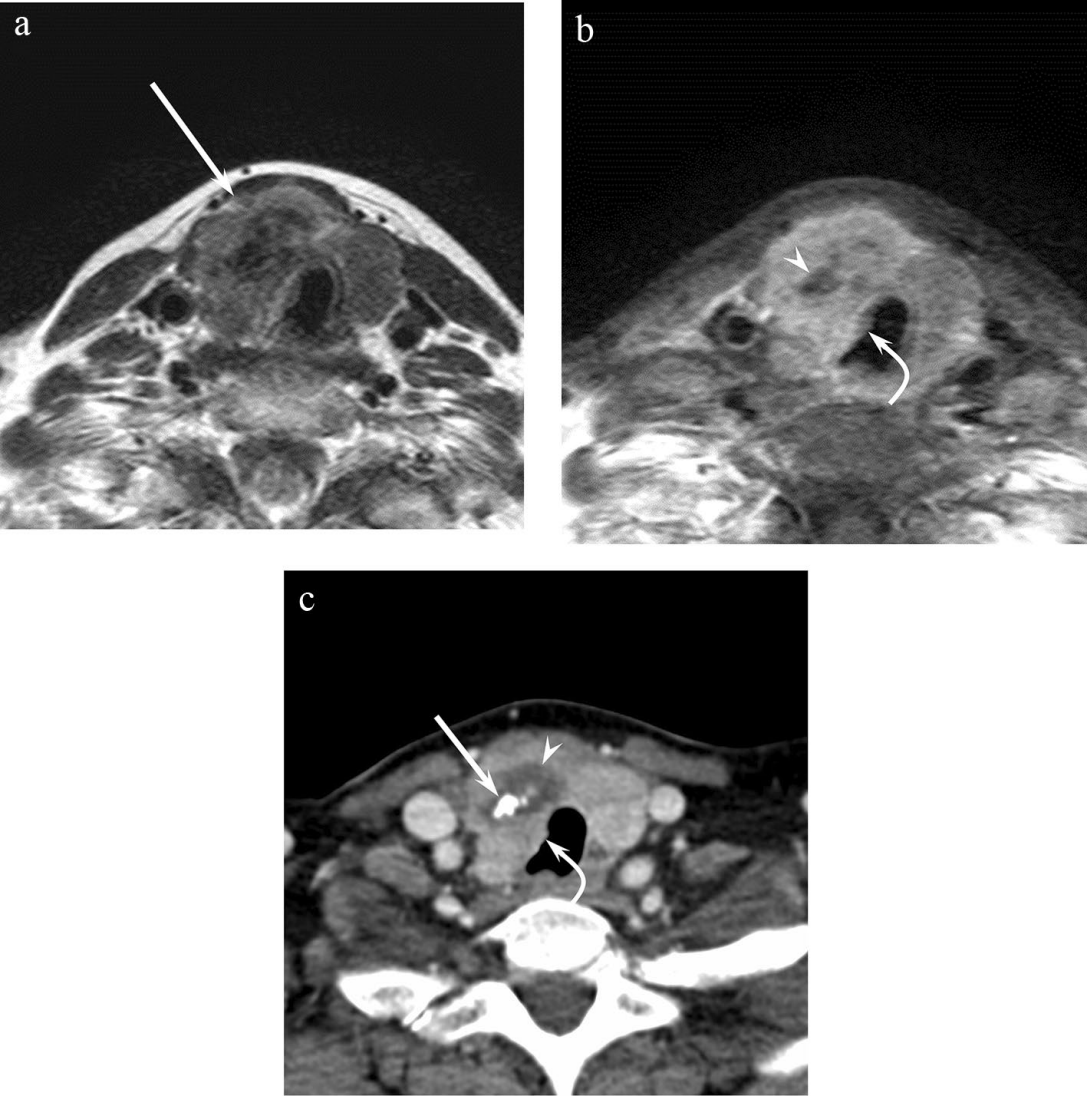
A 64-year-old woman with poorly differentiated thyroid carcinoma of the right lobe. a T2-weighted image (TR/TE, 5710/90 ms) shows a heterogeneously mixed hyper- and hypointense mass (arrow). b Fat-suppressed contrast-enhanced T1-weighted image (TR/TE, 632/15 ms) shows heterogeneous enhancement with unenhanced areas (arrowhead). The tumor shows an ill-defined margin and tracheal invasion (curved arrow). c Contrast-enhanced CT image shows intratumoral nodular calcification (arrow) and unenhanced area (arrowhead). The tumor shows an ill-defined margin and tracheal invasion (curved arrow)
